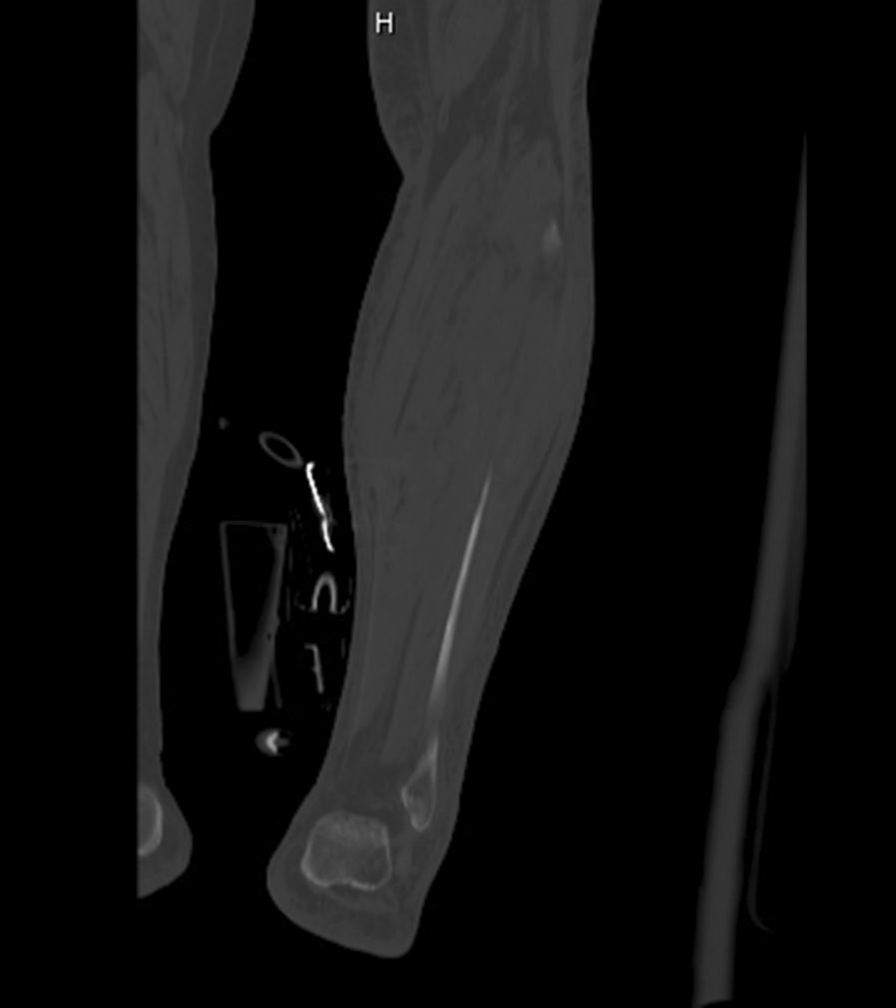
CT sagittal view of the left lower extremity which reveals mild diffuse fatty stranding
